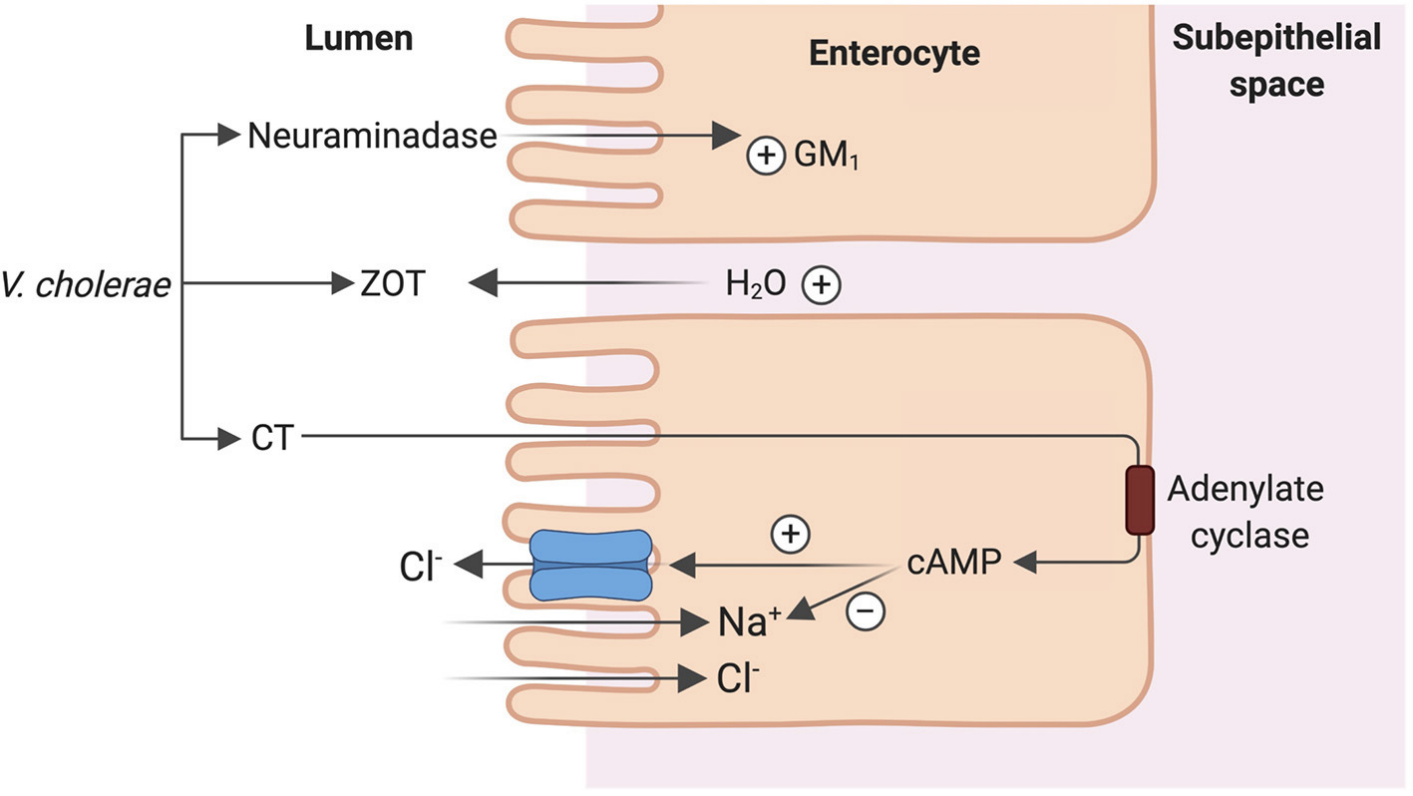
Mechanism of cholera enterotoxicity. *Vibrio cholera* produces 3 toxins that lead to the copious “rice water” cholera diarrhea: cholera toxin (CT), zona occludens toxin (ZOT) and a neuraminidase. CT binding followed by endocytosis leads to increase in cAMP production and ultimately activation of CFTR leading to increased intestinal Cl^−^ secretion and diarrhea. ZOT increases paracellular fluid permeability, contributing to increased stool volume. Neuraminidase increases the number of CT binding sites in adjacent intestinal cells, amplifying the enterotoxicity of CT.
